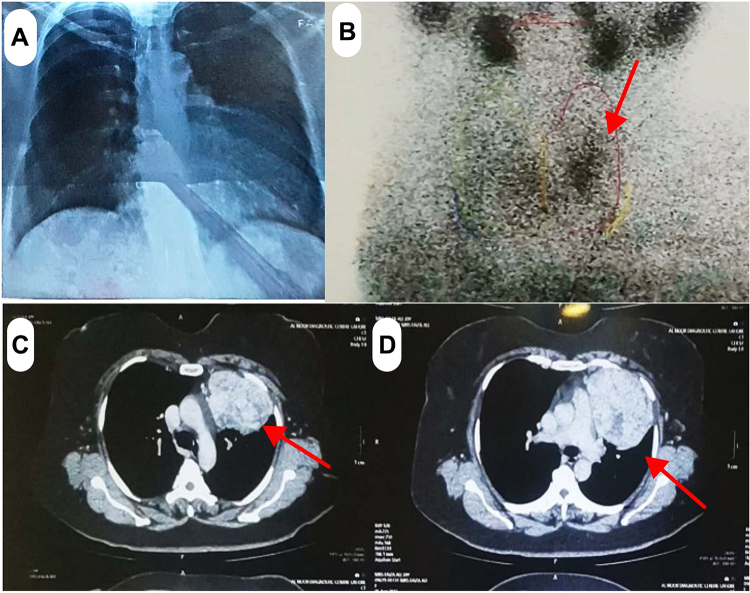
(A) Chest X-ray of the patient. (B) Thyroid scan of the patient. (C) CT chest showing well circumscribed mass. (D) CT chest showing well circumscribed mass.
